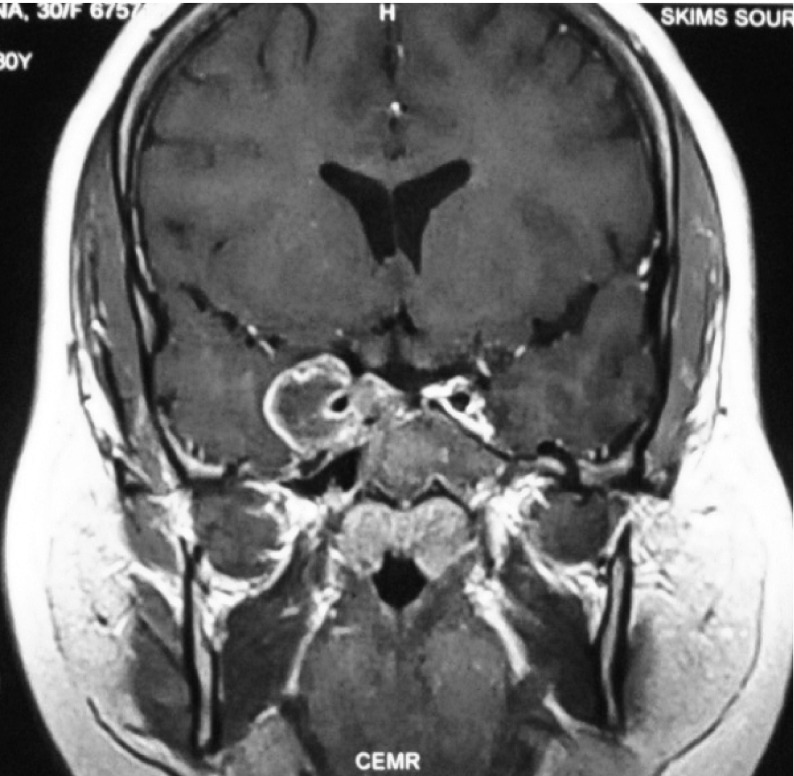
T1 Weighted (Gadolinium enh.) MRI Revealing Large Irregular Sellar Mass With Parasellar Extension With Infiltration of Cavernous Sinus and Encasement of Internal Carotid Artery.
